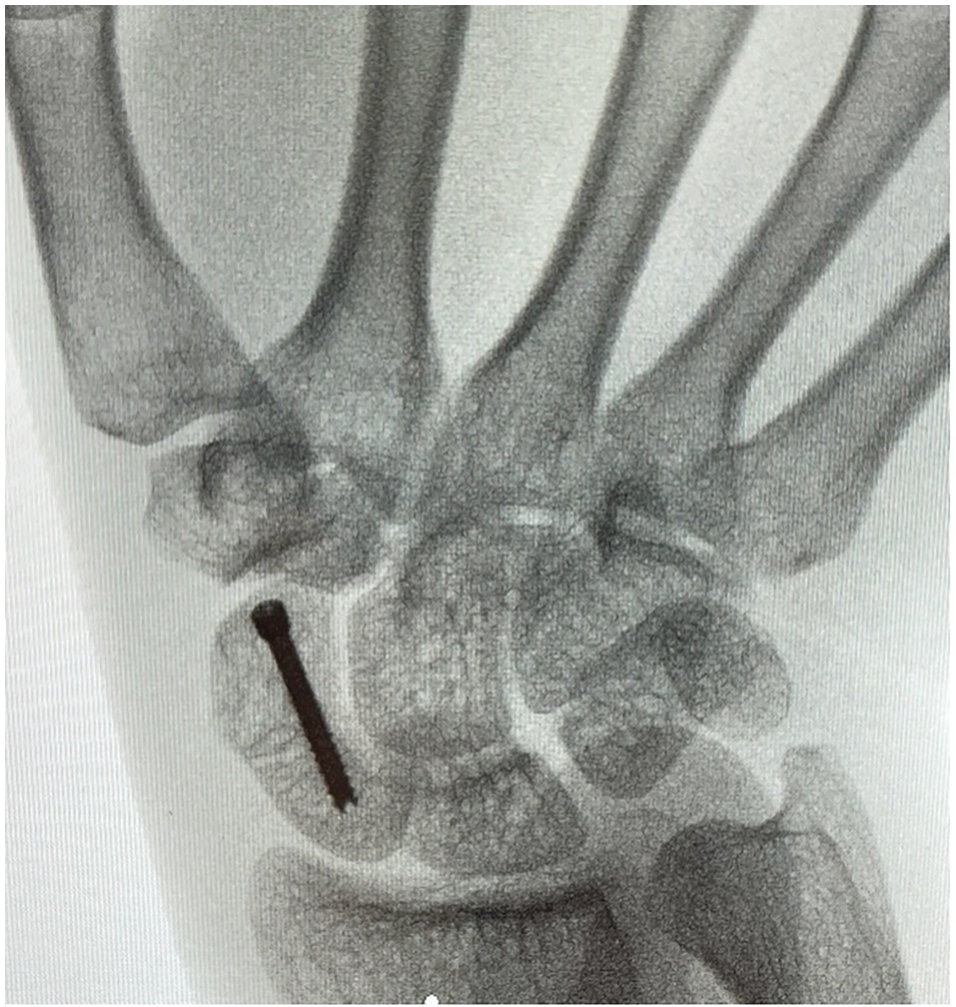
Anterior–posterior X-ray post screw placement.
